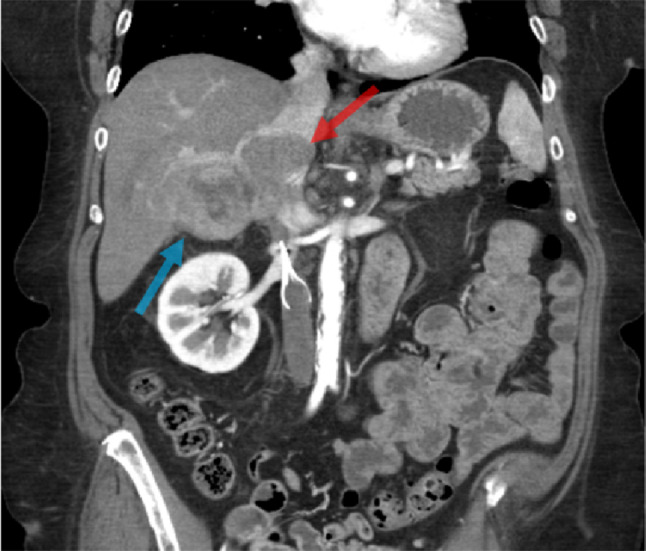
Coronal contrast-enhanced CT image in the arterial phase demonstrates a large bilobed heterogeneously enhancing suprarenal IVC mass with both intravascular (red arrow) and extravascular components (blue arrow). The patient has a IVC filter. Pathology confirmed leiomyosarcoma arising from the IVC
